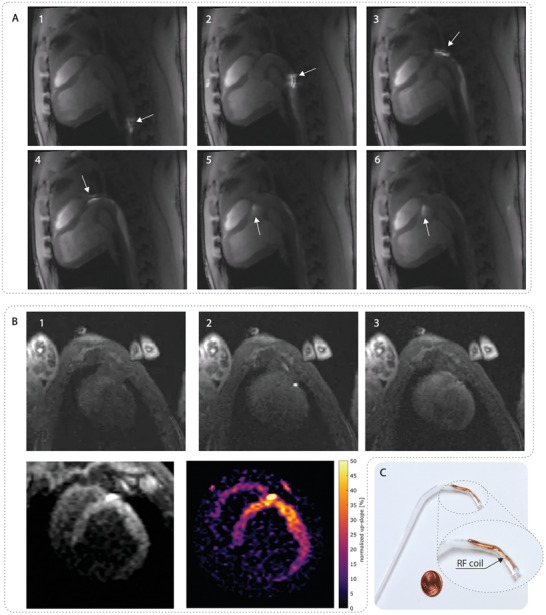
In vivo real‐time MRI of active tracking catheter and photograph of tip details. A) Catheter crossing the aortic arch with arrows pointing at the catheter tip. B) Intracoronary injection of gadolinium‐containing contrast for selective perfusion, enabling semi‐quantitative mapping by normalizing values to the up‐slope in the left anterior descending artery. C) Active tracking catheter tip with labeled positions of RF coil.
